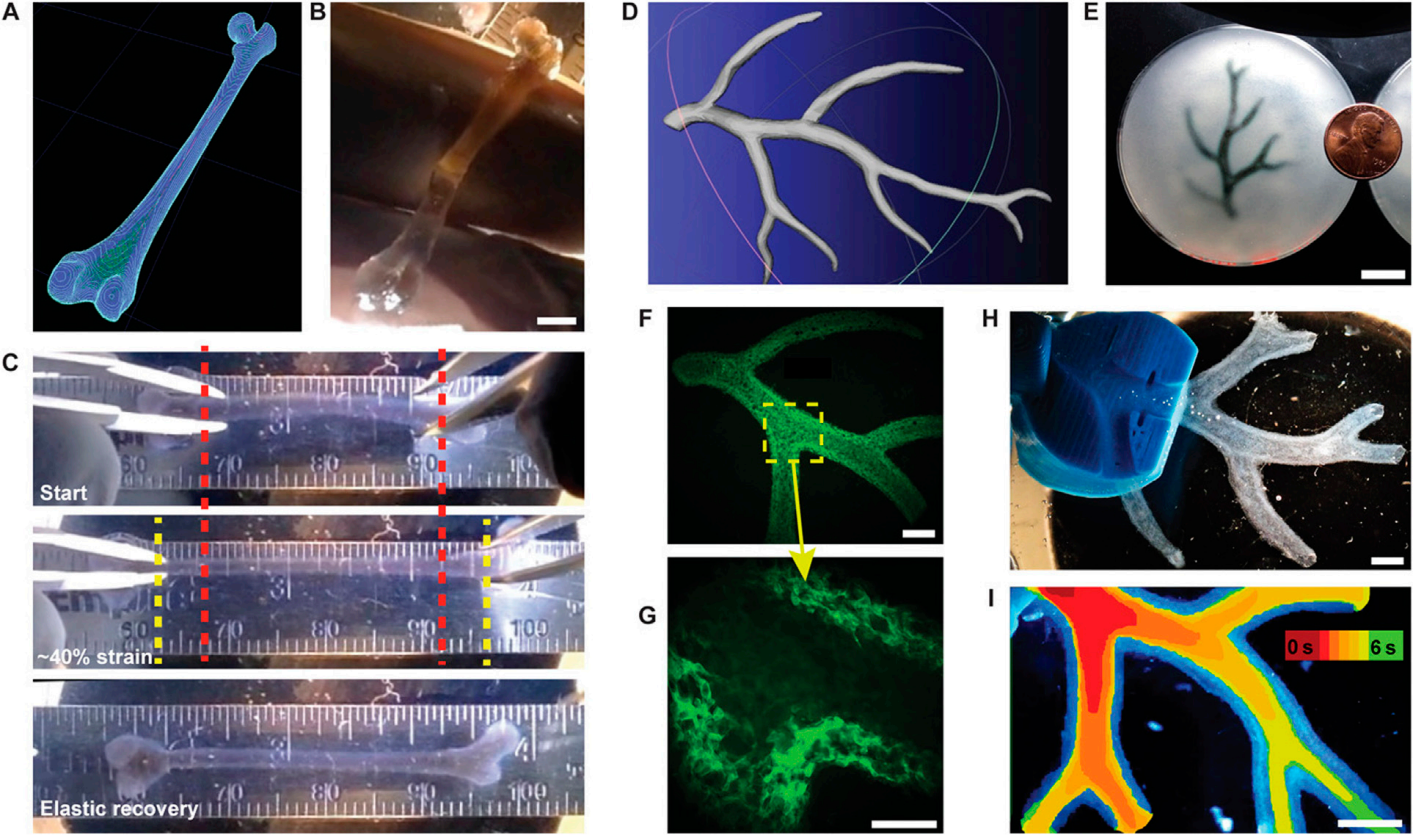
FRESH printing of biological structures based on 3D imaging data and functional analysis of the printed parts. (A) A model of a human femur from 3D CT imaging data is scaled down and processed into machine code for FRESH printing. (B) The femur is FRESH printed in alginate, and after removal from the support bath, it closely resembles the model and is easily handled. (C) Uniaxial tensile testing of the printed femur demonstrates the ability to be strained up to 40% and elastically recover. (D) A model of a section of a human right coronary arterial tree from 3D MRI is processed at full scale into machine code for FRESH printing. (E) An example of the arterial tree printed in alginate (black) and embedded in the gelatin slurry support bath. (F) A section of the arterial trees printed in fluorescent alginate (green) and imaged in 3D to show the hollow lumen and multiple bifurcations. (G) A zoomed-in view of the arterial tree shows the defined vessel wall that is < 1 mm thick and the well-formed lumen. (H) A dark-field image of the arterial tree mounted in a perfusion fixture to position a syringe in the root of the tree. (I) A time-lapse image of black dye perfused through the arterial tree false-coloured at time points of 0–6 s to show flow through the lumen and not through the vessel wall. Scale bars, 4 mm (B), 10 mm (E), 2.5 mm (F), 1 mm (G), and 2.5 mm (H, I). Adopted with permission from Hinton et al. (2015).
